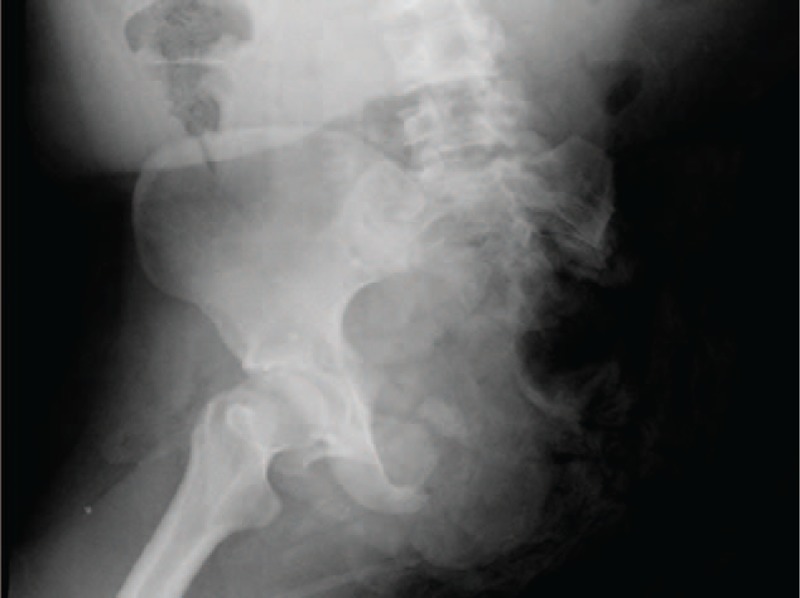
Initial radiograph after injury.
